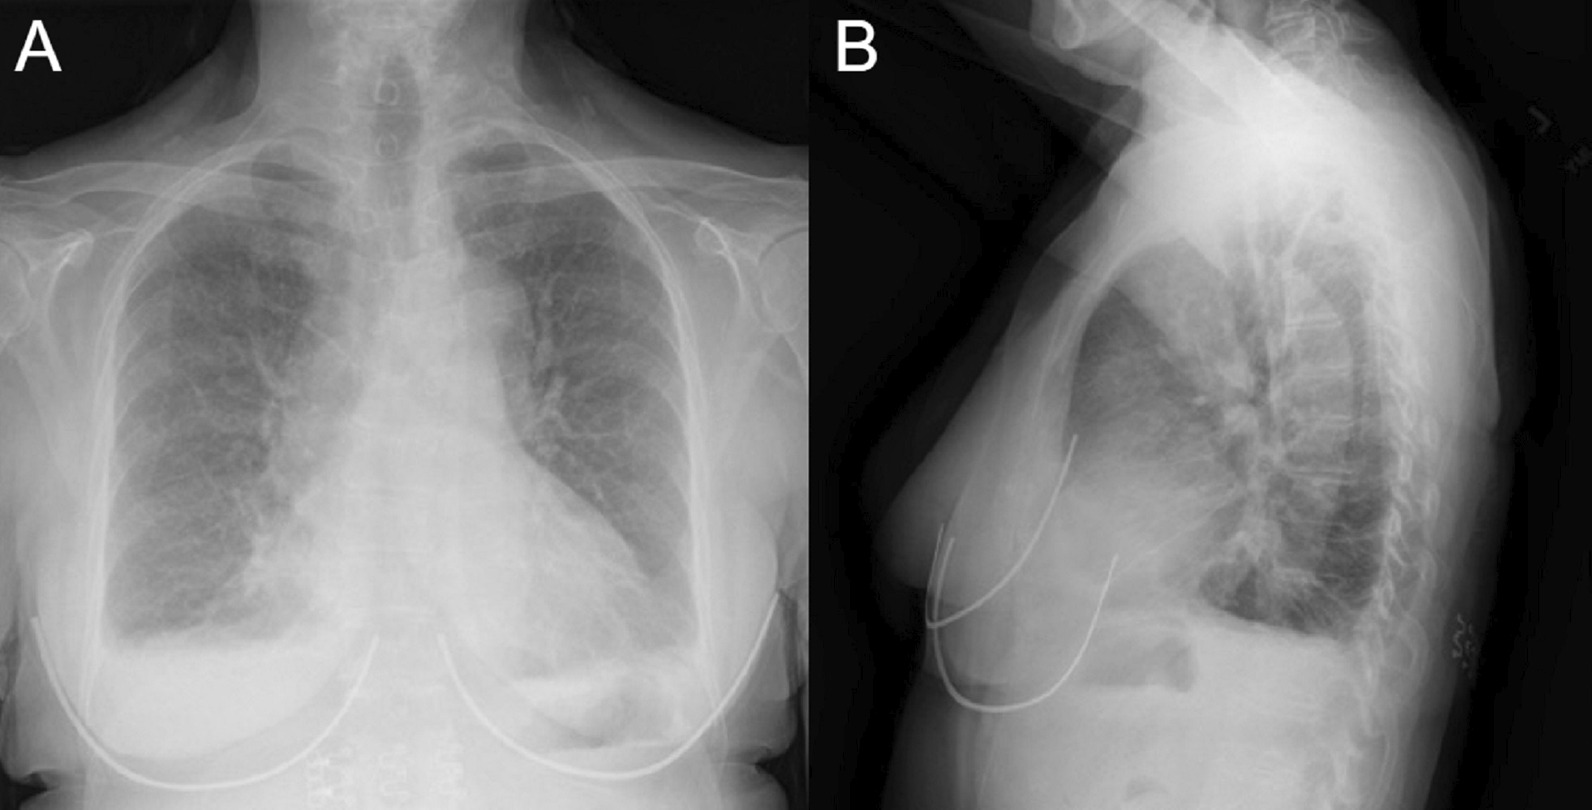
Case 1. Posteroanterior A and lateral B chest X-ray. Enlarged cardiomediastinal silhouette and small bilateral pleural effusions
